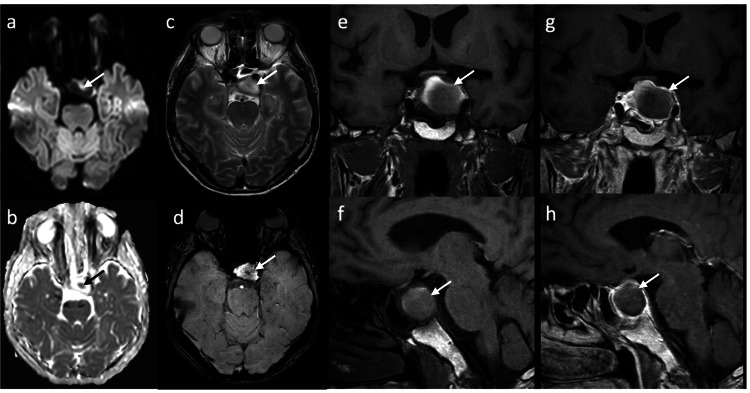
Follow-up MRI eight days after initial CT shows a central area of diffusion restriction (arrow) on axial diffusion-weighted images (a and b). The mass (arrow) is relatively hyperintense on axial T2-weighted images (c) and shows a central susceptibility artefact compatible with hemorrhage or infarction on axial susceptibility-weighted images (d). T1-weighted coronal (e) and sagittal (f) images show incomplete peripheral hyperintensity. After contrast administration, the peripheral enhancement appears slightly less pronounced on coronal (g) and sagittal (h) T1-weighted images than on the initial MRI. CT: computed tomography, MRI: magnetic resonance imaging.
